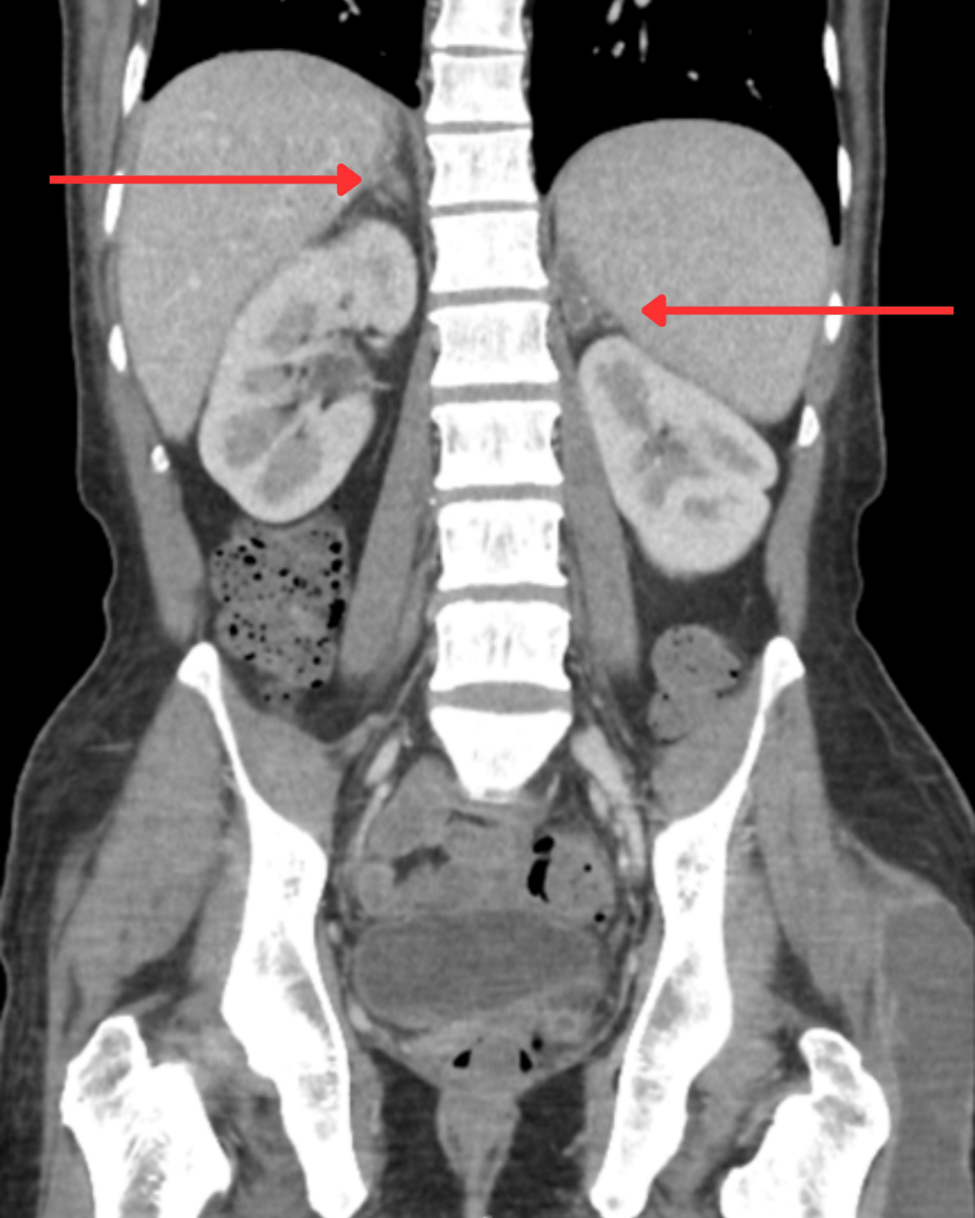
Follow-up CT adrenal glands demonstrating features of adrenal TB indicated by red arrows.
